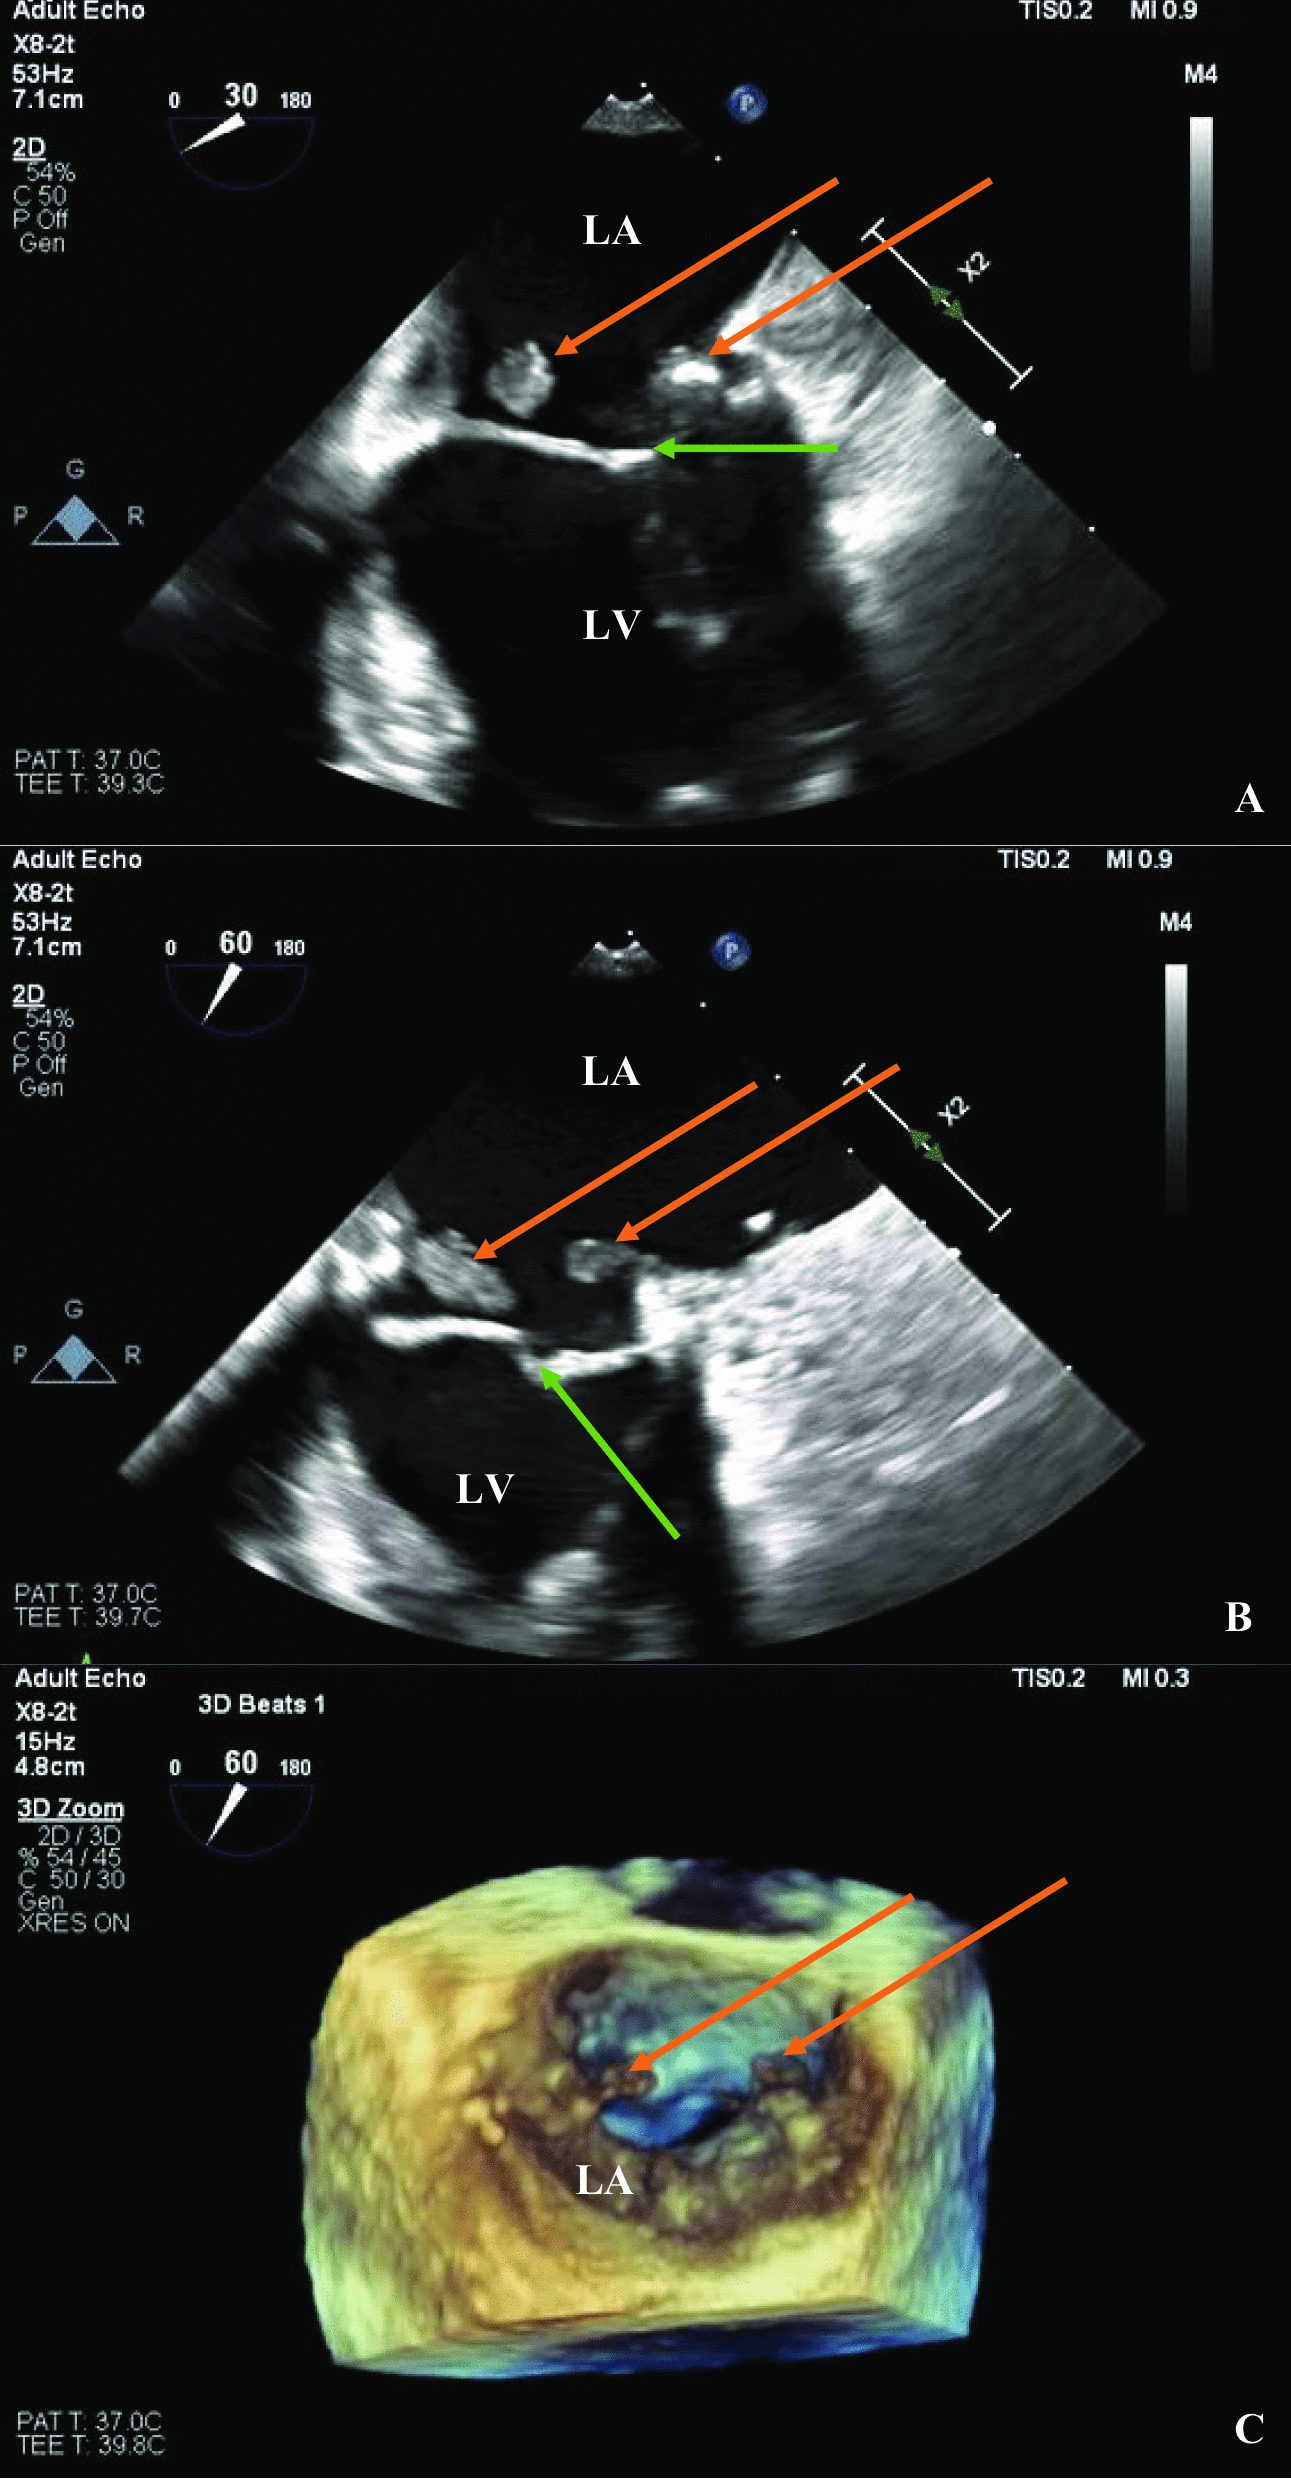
Transesophageal echocardiography images. A, B Transesophageal echocardiography at the mitral valve commissural view at 30° and 60°, respectively, with the two mitral annular masses shown by orange arrows and the mitral valve with green arrows. C Transesophageal echocardiography from the mid-esophageal short axis position revealing the three-dimensional “surgeon’s view.” The masses again shown with orange arrows. The left ventricle is denoted LV
